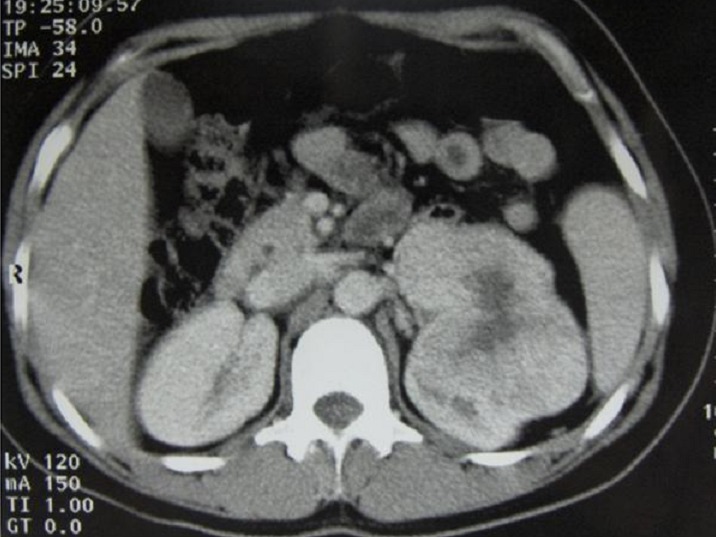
Computed tomography, shows a left renal tumor with central stellate scar
